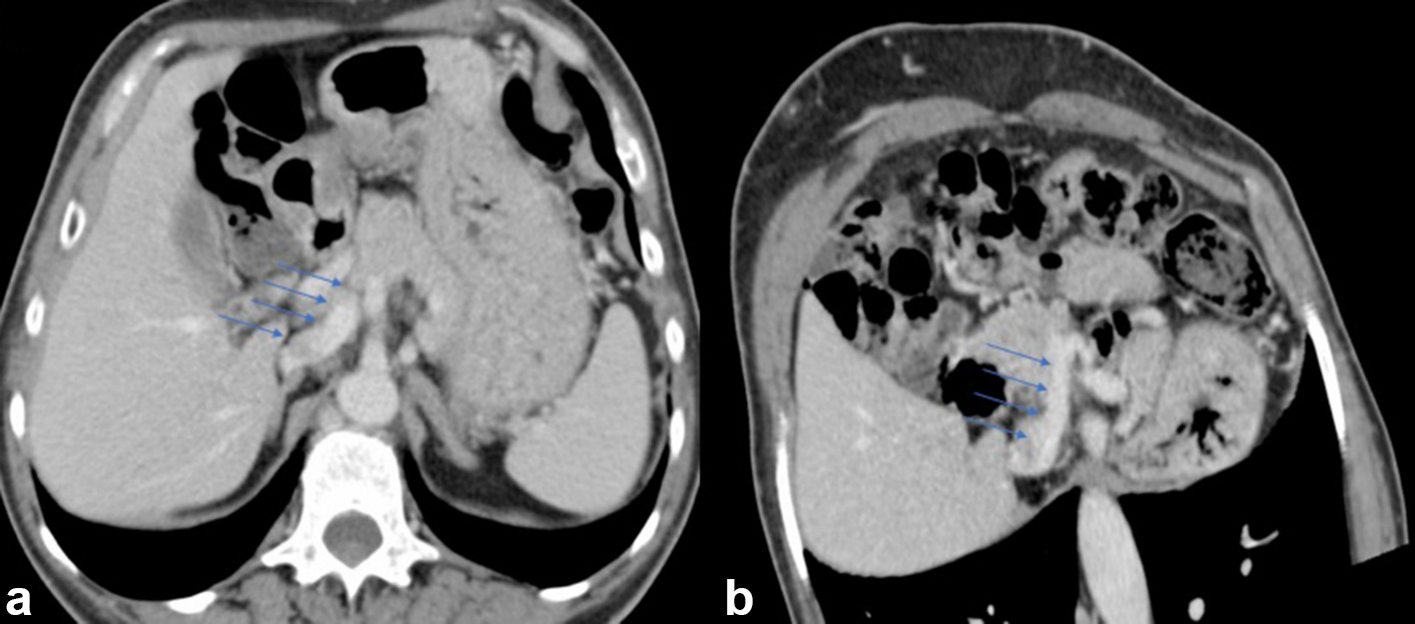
Multiplanar curved reformatted CT images better depicted the short abnormal portal vein draining in the inferior vena cava (a and b).
